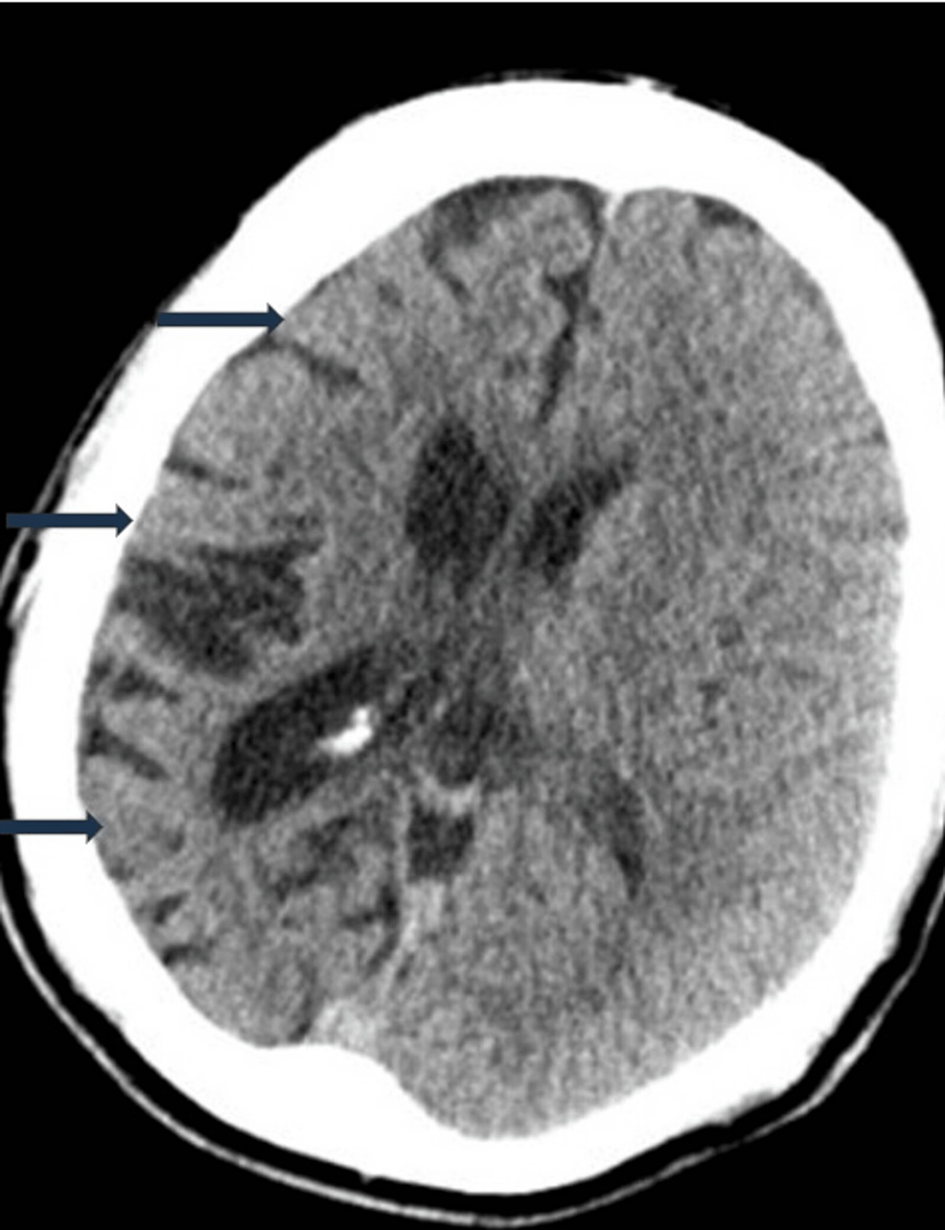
Transverse section of a non-contrast CT of the head revealing a disproportionate atrophy of the right cerebral hemisphere and associated prominence of the sulci and ipsilateral lateral ventricle.
